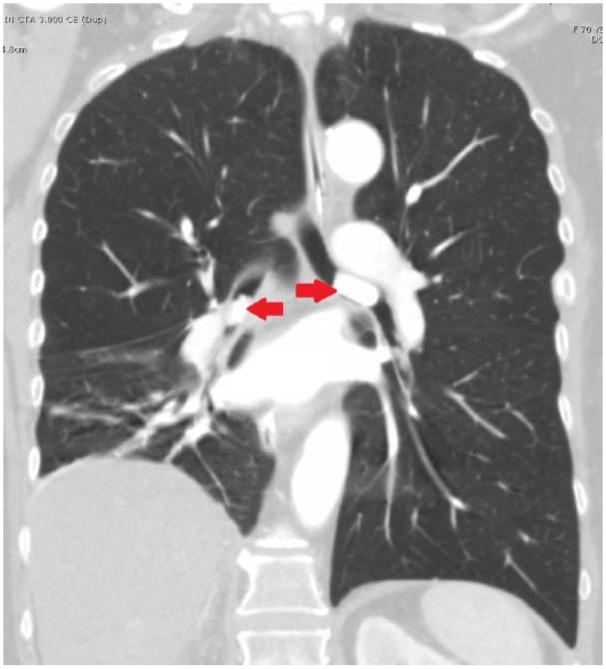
Coronal view of computed tomography scan of thorax showing calcified foreign objects (red arrows) in the left and right main bronchus.
